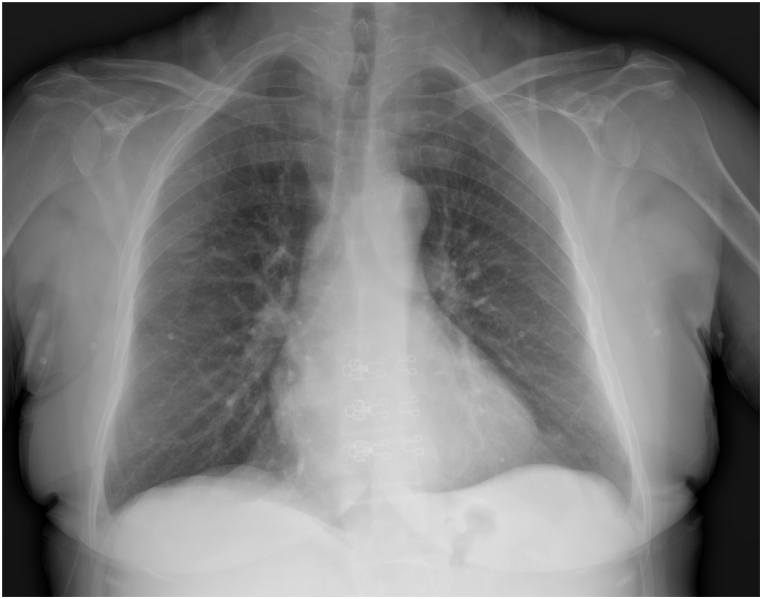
Preoperative chest radiograph demonstrating clear lung fields and absence of pleural effusion.
